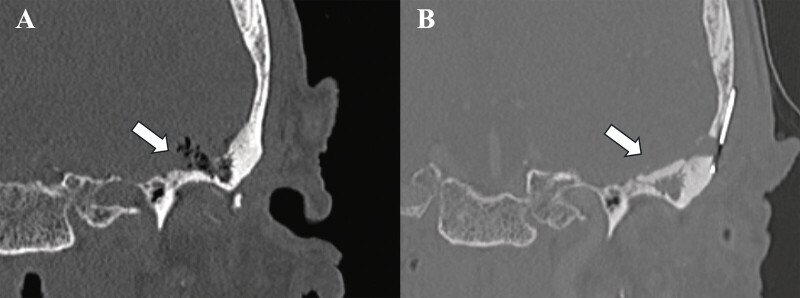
Case 1 coronal CT images before (
